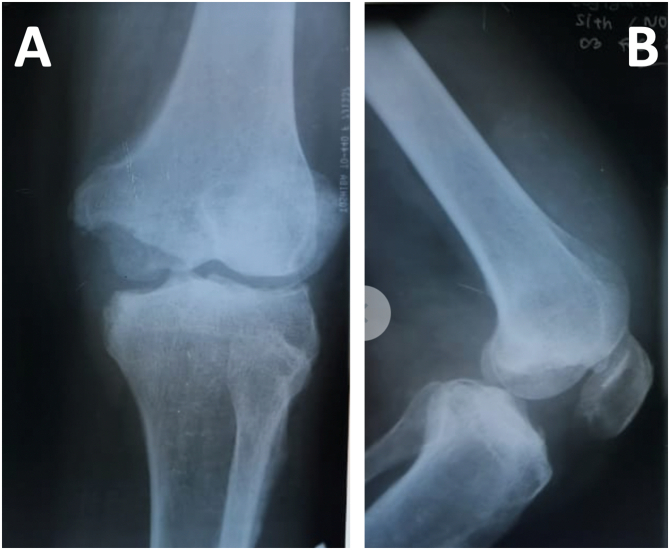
Preoperative Left Knee Radiograph Antero Posterior and Lateral View juxta-articular osteoporosis, peripherally distributed osseous erosions, joint space narrowing, and medial femoral condyle bony defect (A)(B).
